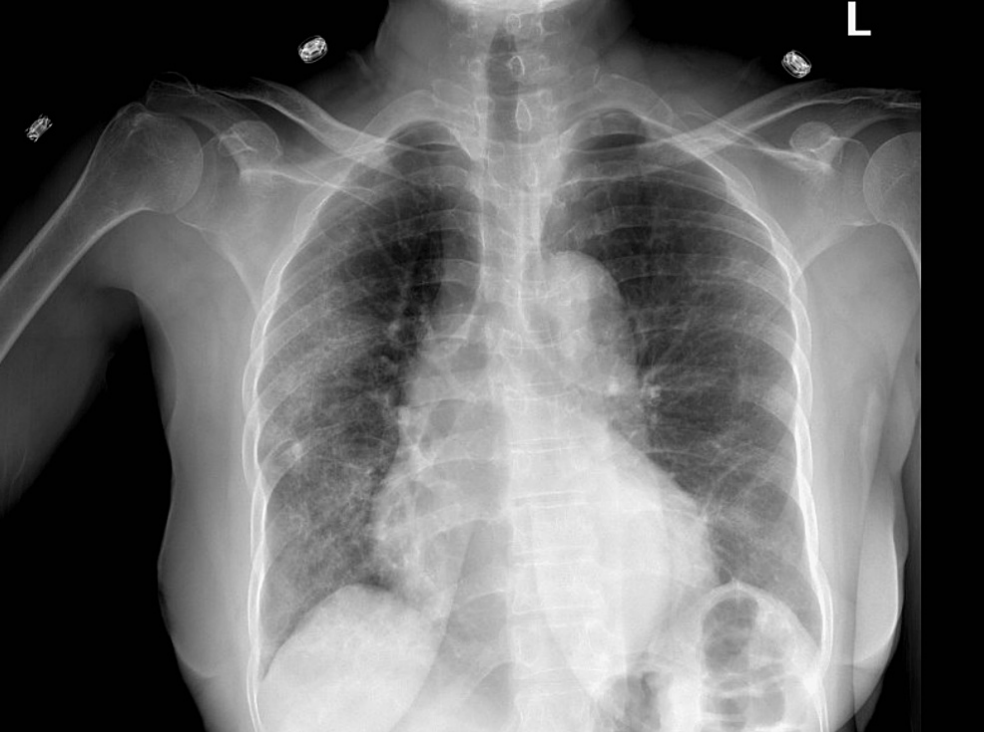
Chest X-ray showing cardiomegaly
